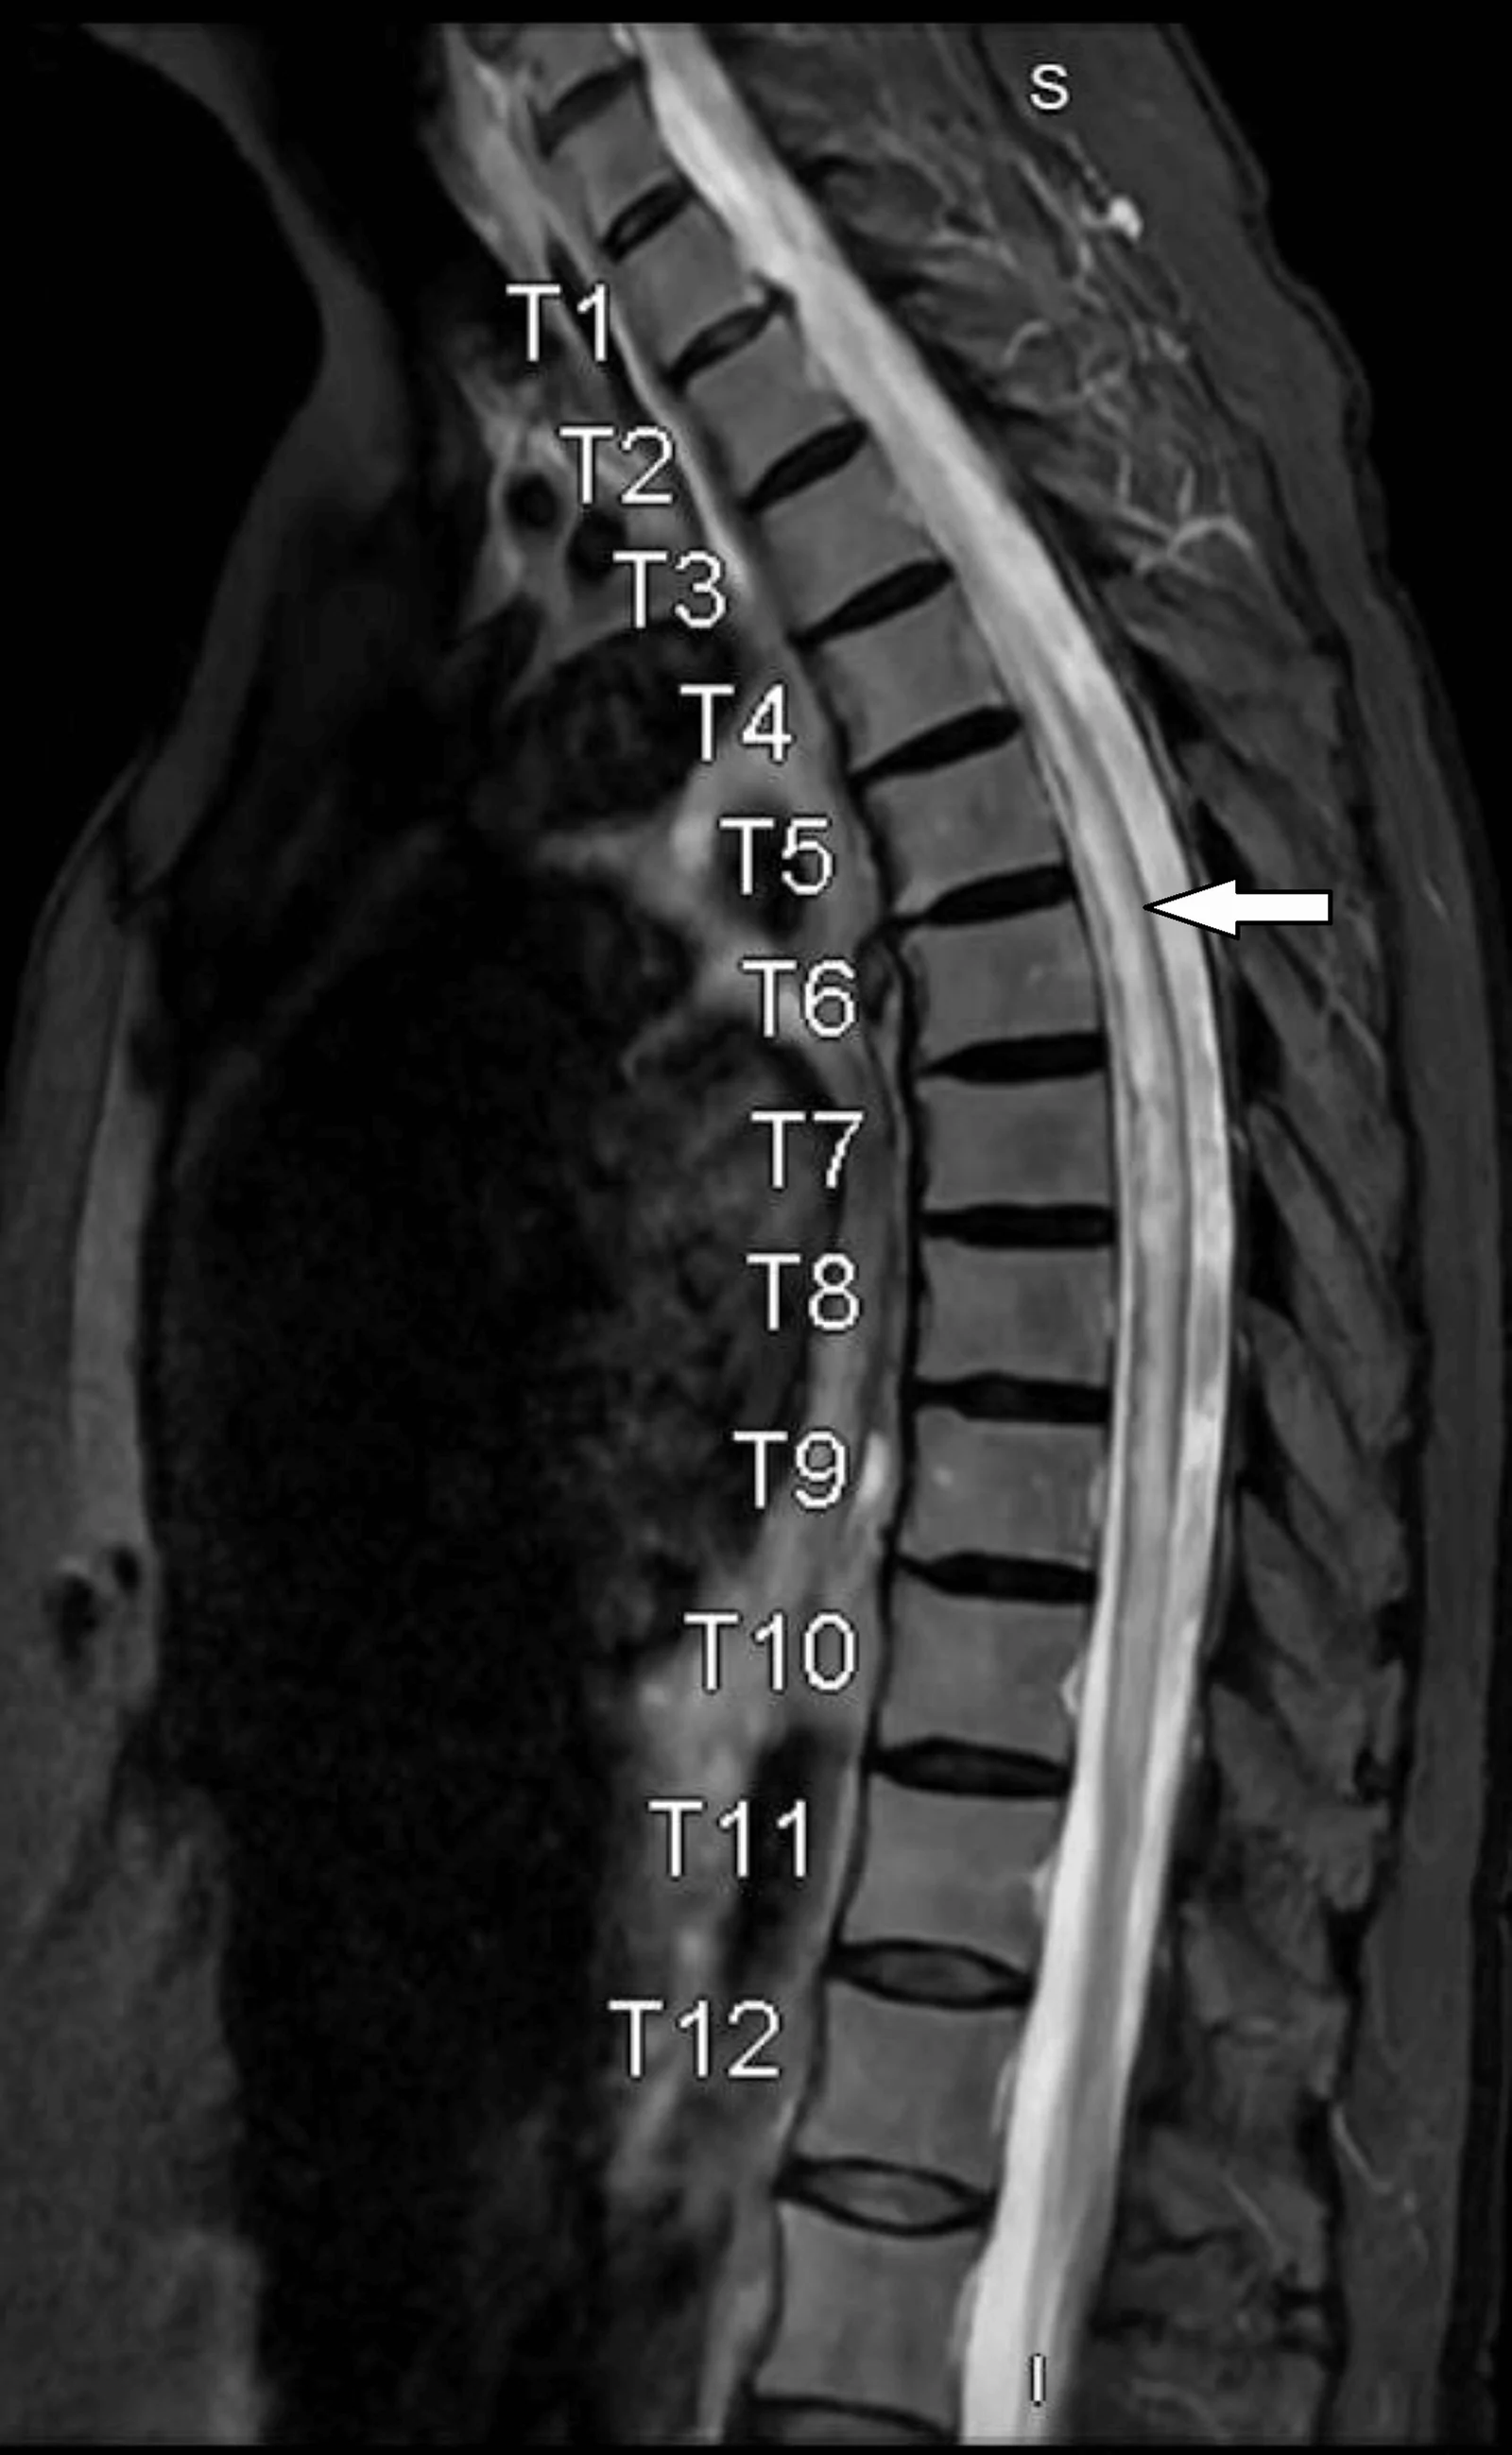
MRI T2-STIR showing extensive T2 signal abnormalities involving the central cord spanning T2-T11, with signal abnormalities spanning T4-T9.
